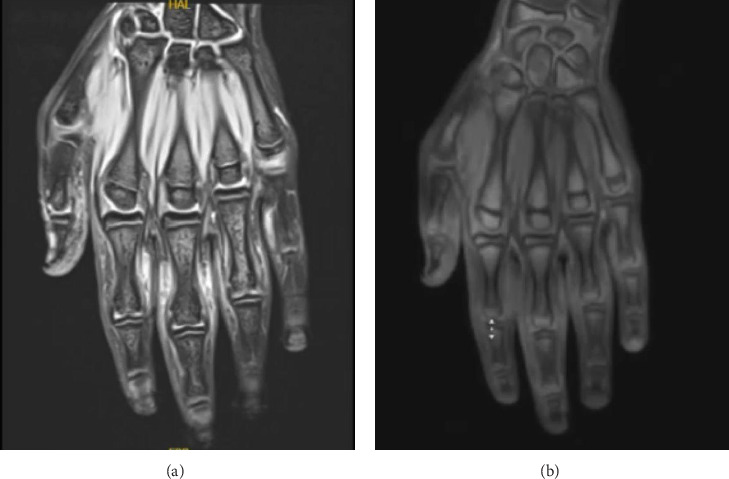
Coronal views (a-b) of magnetic resonance imaging of the left hand reveal an oval-shaped subcutaneous soft tissue mass, characterized by T1 hypointensity and minimally enhancing T2 hyperintensity, with no evidence of joint effusion or synovitis.
